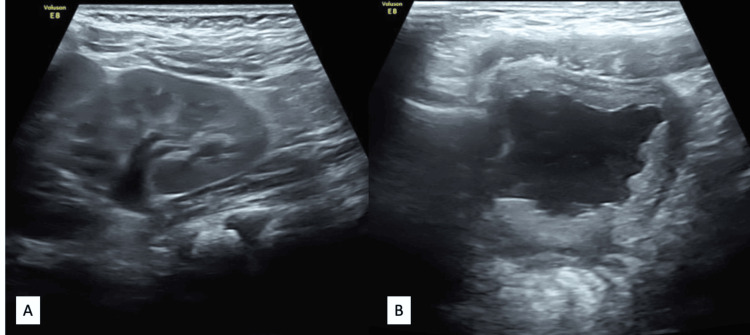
Ultrasound images of (A) the left kidney showing mild hydronephrosis and (B) the bladder showing diffuse wall thickening, trabeculations, and low level internal echoes in the bladder lumen
